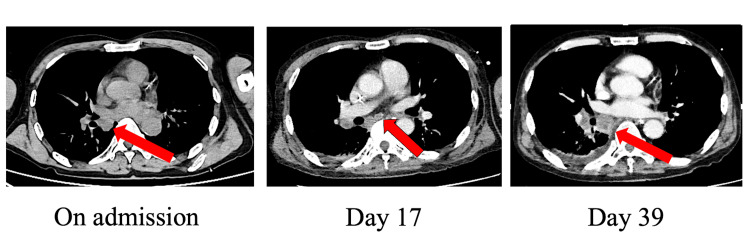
CT scan on admission and day 17 and day 39 of hospitalization: findings of lung tumor (red arrow) are gradually increasing in size
